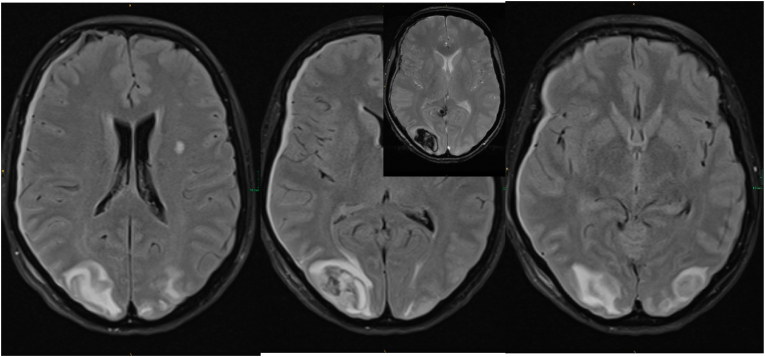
Follow up MRI (three subsequent FLAIR weighted images from cranial to caudal with a corresponding T2∗ section in the upper left corner of the middle image) again 24h later showing both, significant white matter hyperintensity reflecting vasogenic edema and subacute hematoma.
